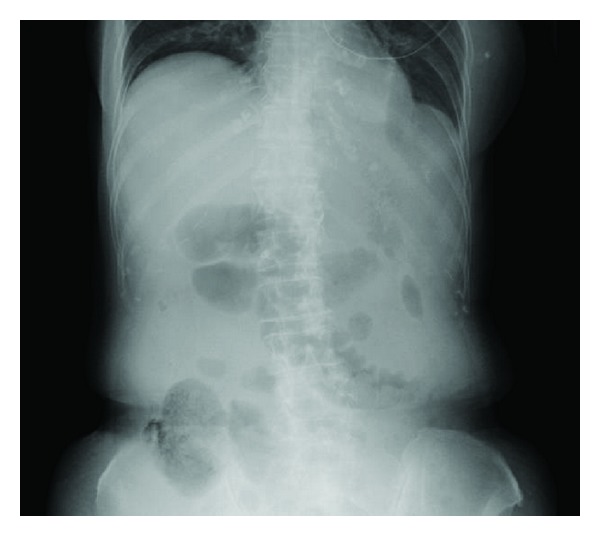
Plain abdominal X-ray film. No free intraperitoneal gas was observed.
